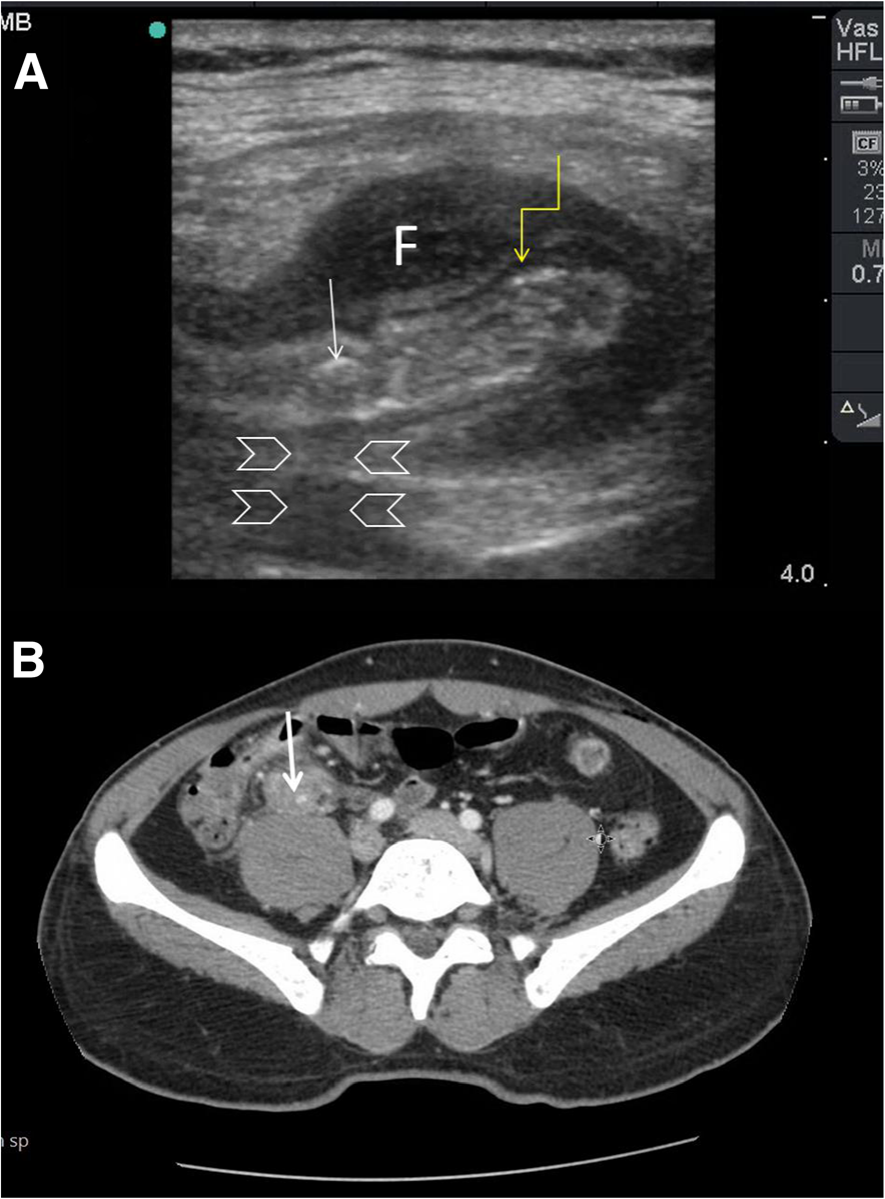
A 40-year-old man presented complaining of fever and pain in the right iliac fossa of 5 days duration. A hard tender mass was felt in the right iliac fossa. CRP was high. POCUS study (a) using a portable ultrasound machine and a high-frequency linear probe has shown a thickened appendicitis with oedema in the muscularis propria (yellow arrow) surrounded by fluid (F). There was a faecolith (white arrow) with a shadow behind it (arrowheads). Abdominal CT scan with intravenous contrast has confirmed the same findings (b). Interval appendectomy which was performed two months later confirmed the diagnosis
